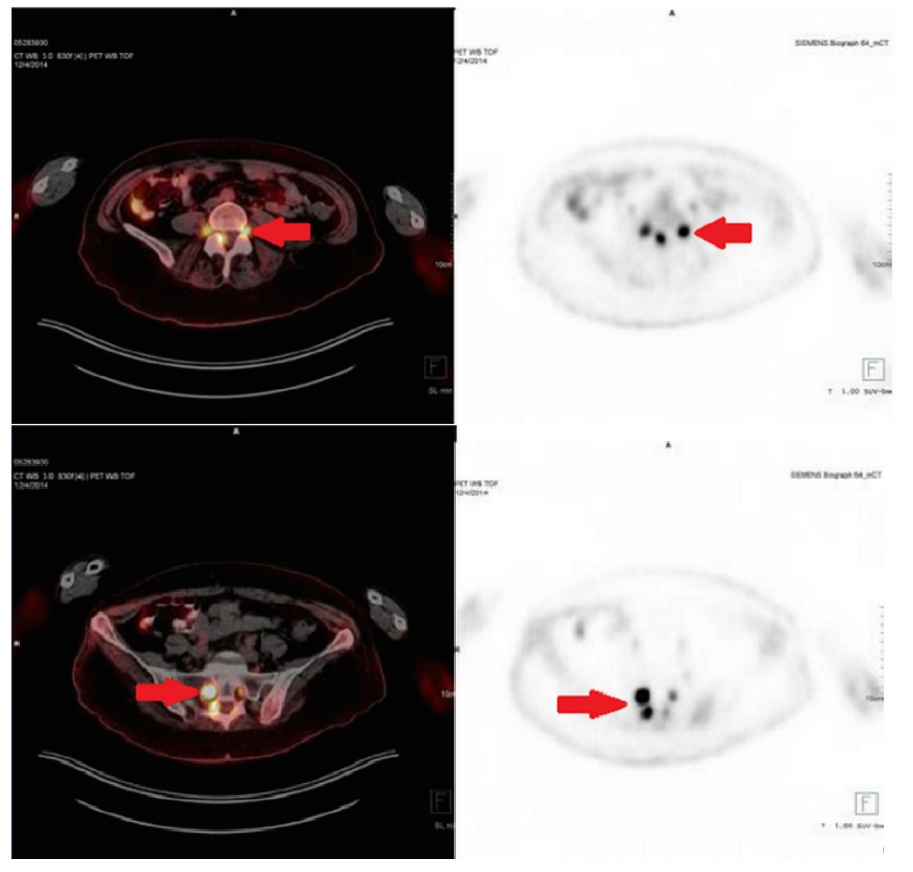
FDG-PET/CT for recurrence assessment FDG-avid involvement of the left T1, bilateral lumbar and sacral nerve roots as well as the right sacral plexus and right sciatic nerve (see arrows).
